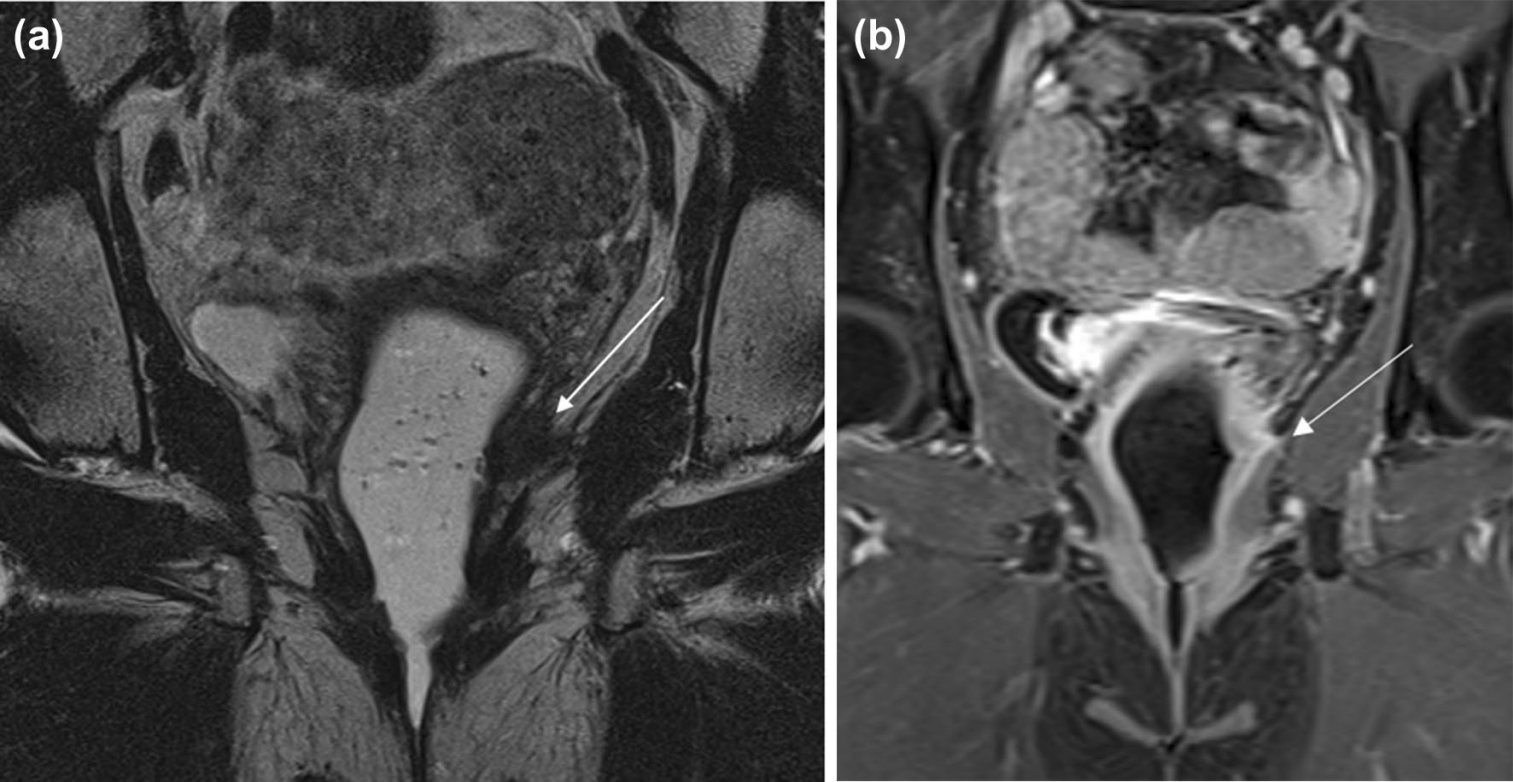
51-year-old woman with FIGO stage 2 squamous cell carcinoma of the vagina. a Coronal oblique long-axis T2-weighted image through the vagina shows a hypointense mass arising from the left vaginal wall (arrow) and extending into the paravaginal soft tissue. b Coronal T1-weighted post-contrast image shows enhancement within the paravaginal soft tissue (arrow)
